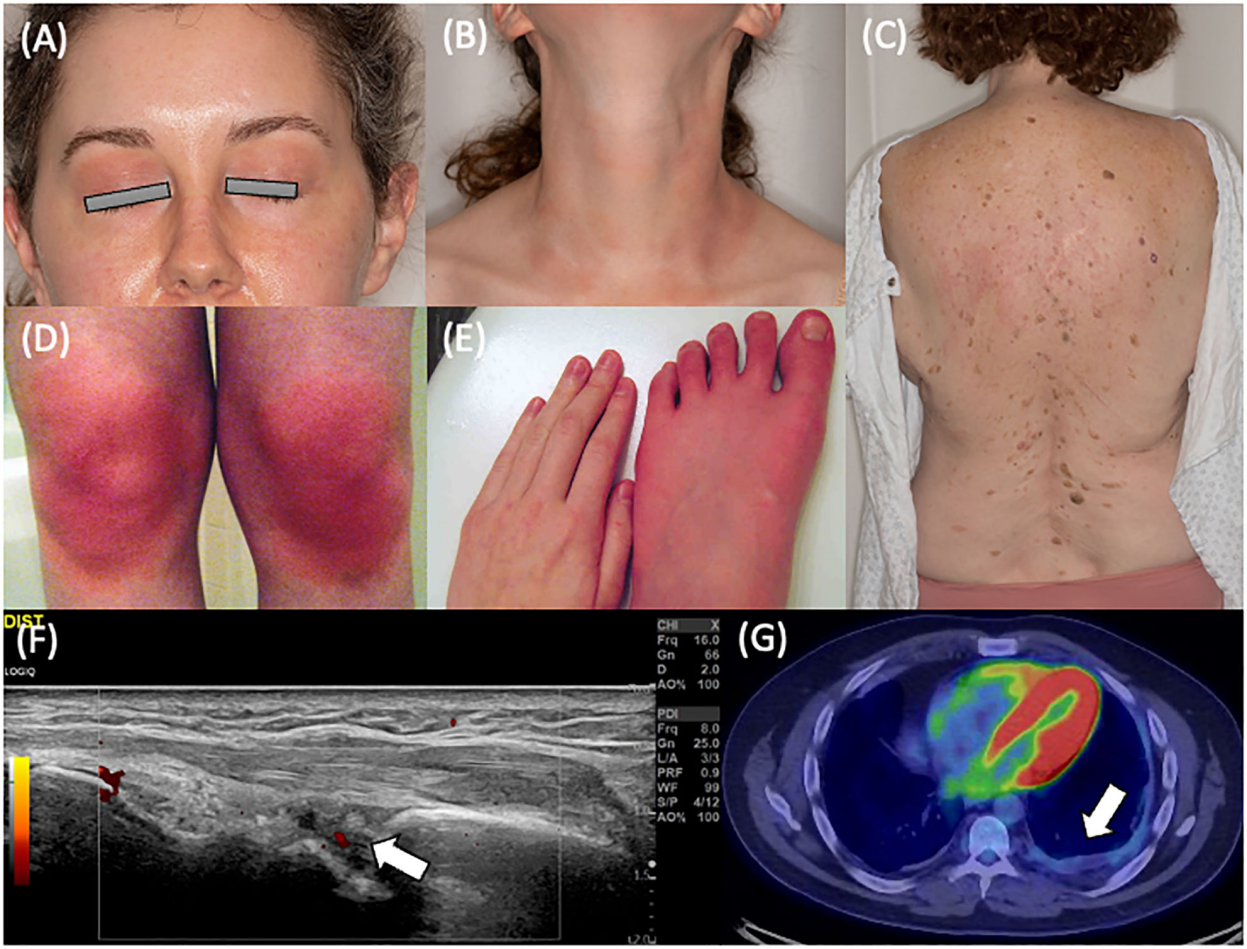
Clinical features of YAOS in the Mayo Clinic cohort. (A) Eyelid redness and swelling and (B) erythematous patches and plaques on the anterior neck of a patient with *NOD2* IVS8 + 158 and R702W variants. (C) Erythematous maculopapular eruption on the back of a patient with *NOD2* IVS8 + 158 and R702W variants. Dermatopathology revealed focal follicular mucinosis and mild perivascular lymphocytic inflammation. (D) Cutaneous flushing overlying the bilateral knees and (E) distal extremity swelling and erythema in a patient with *NOD2* IVS8 + 158 and R702W variants. (F) Musculoskeletal ultrasonography of the dorsal radiocarpal joint in longitudinal scan, demonstrating grayscale and power Doppler synovitis in a patient with *NOD2* IVS8 + 158 variant. (G) Positron emission tomography showing left basilar pleural thickening with faint fluorodeoxyglucose avidity in a patient with restrictive lung disease in a patient with two copies of NOD2 IVS8 + 158, R702W, and 1007fs variants.
